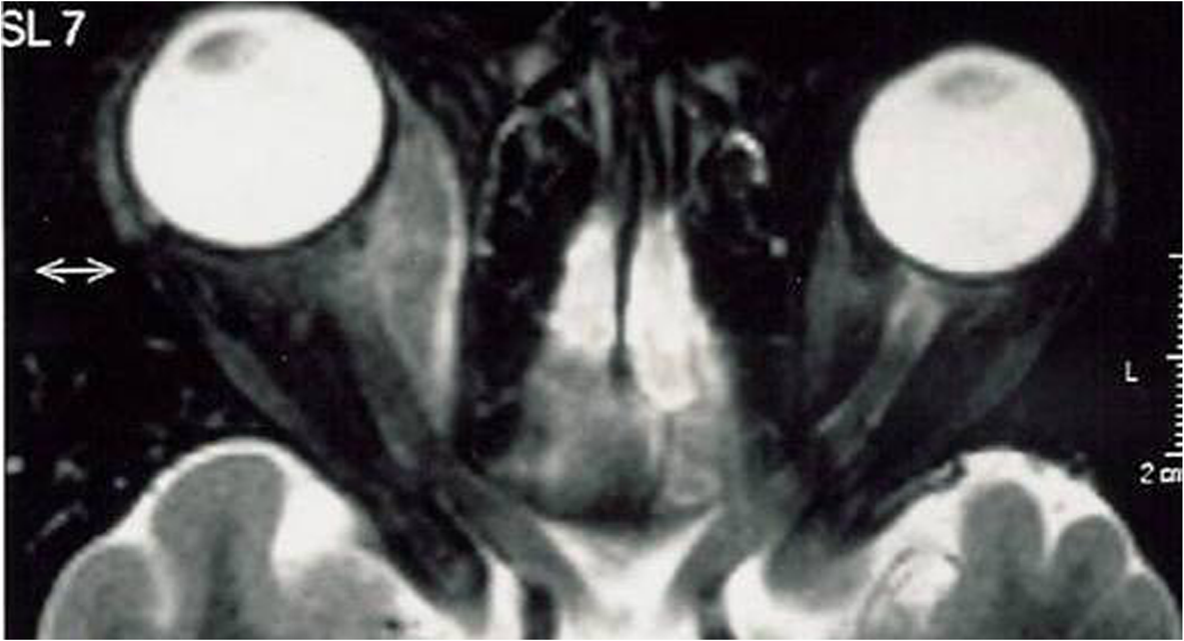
Computed tomography image showed enlargement of medial rectus muscle and proptosis in the right eye (bidirectional arrow).
